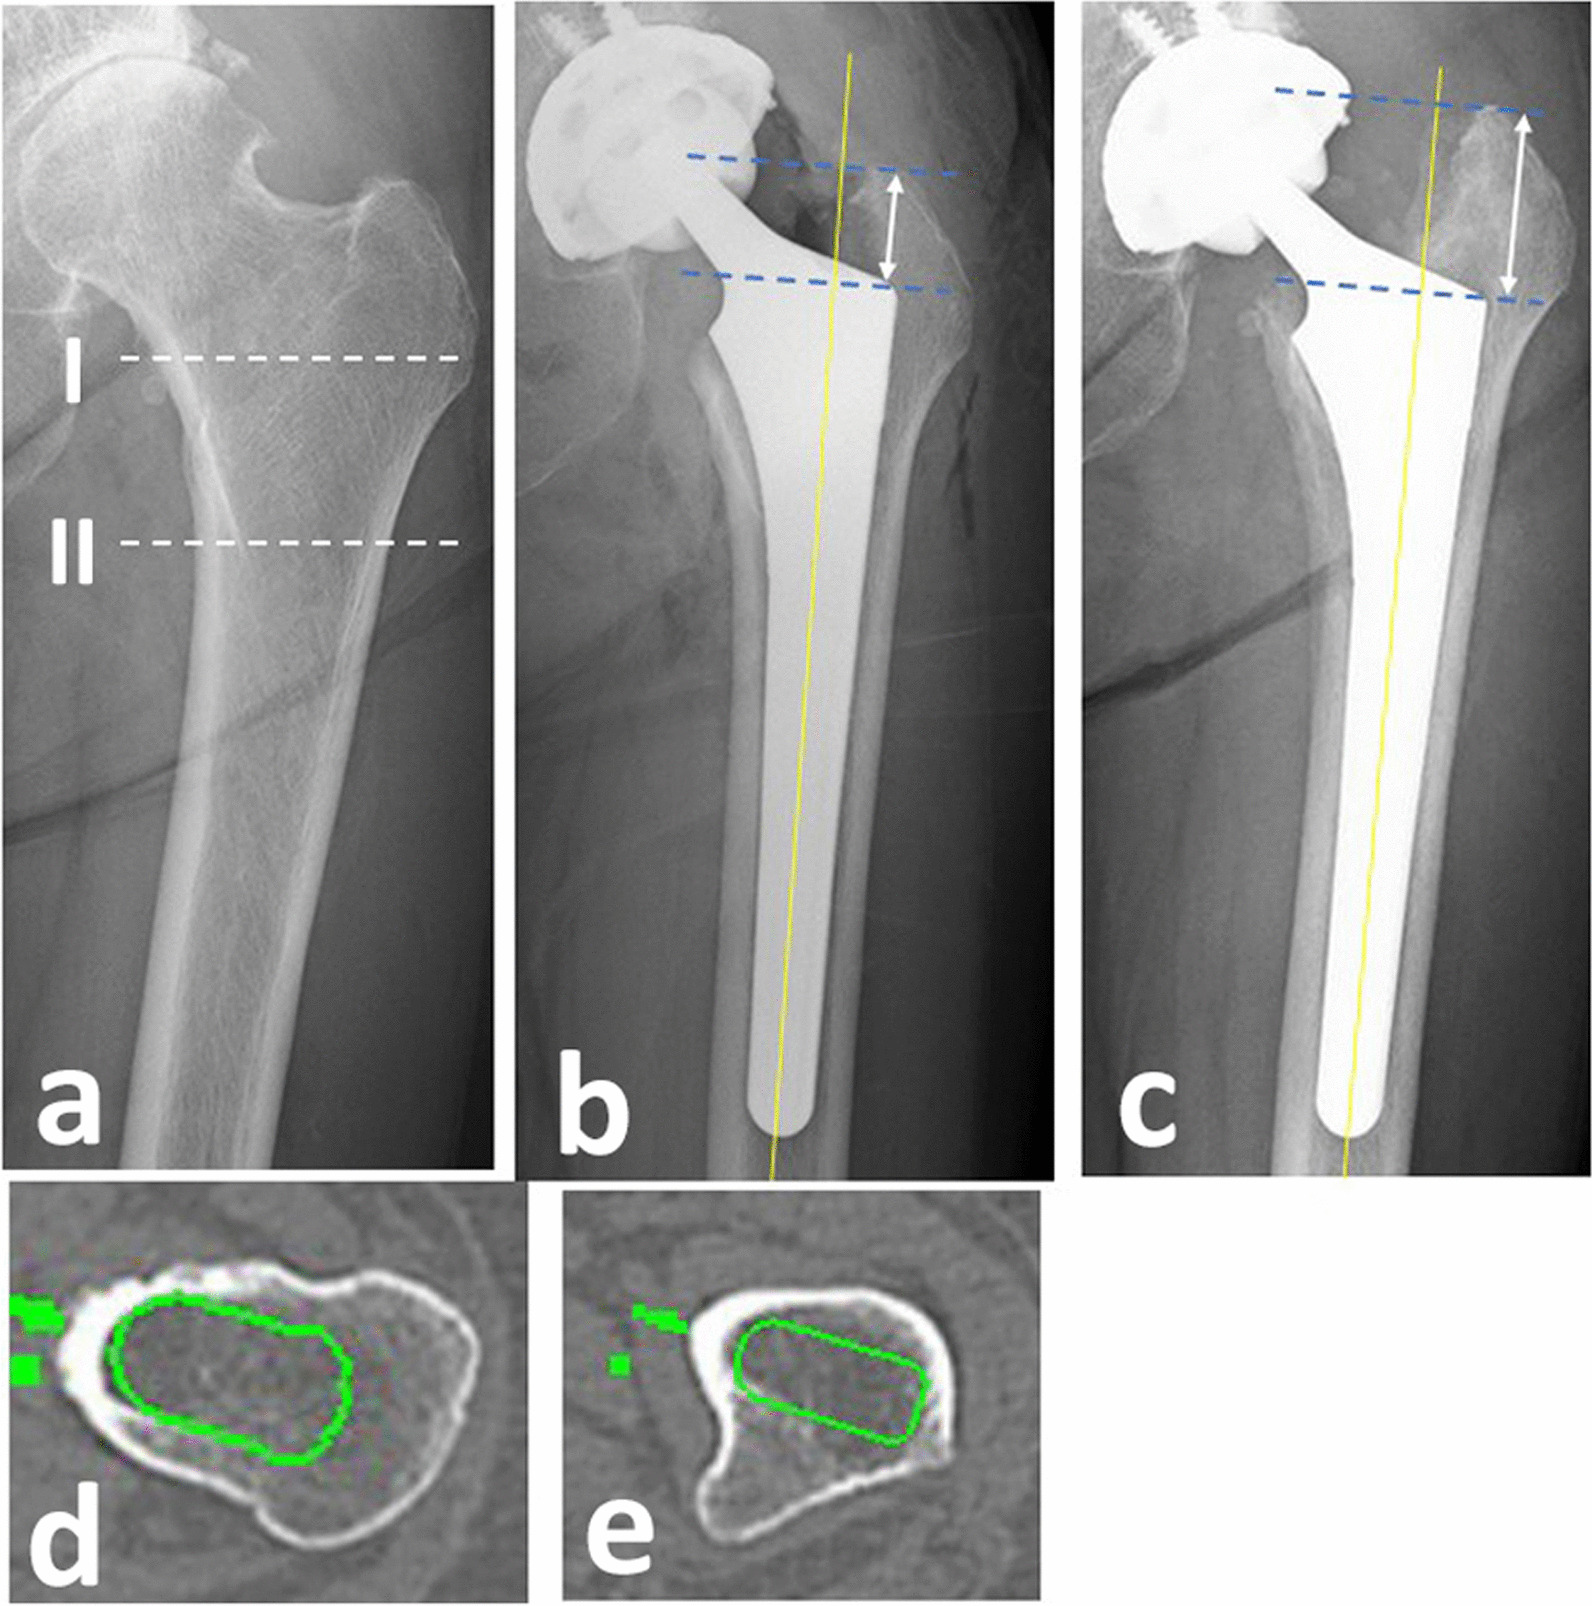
a Left hip joint radiograph of a 78-year-old female with advanced osteoarthritis. (I) The level of fixation of fit-and-fill stem, (II) The level of fixation of tapered wedge stem. b Patient was treated by cementless total hip arthroplasty (THA) using fit-and-fill stem. c The degree of stem subsidence is 8.2 mm 6 weeks after the surgery. d Preoperative three-dimensional (3D) planning (axial plane) for fit-and-fill stem (3D template, Kyocera, Kyoto, Japan) at the level of I in a. e Preoperative 3D planning (axial plane) for tapered wedge stem at the level of II in a
